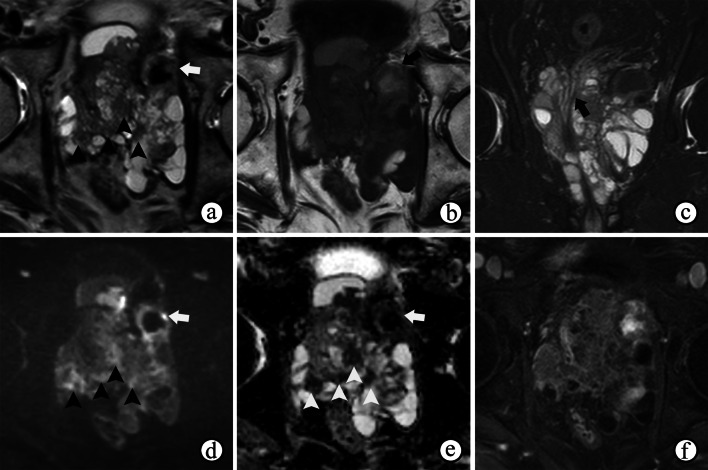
Mixed ductal and acinar adenocarcinoma in an 87-year-old man with dysuria for 12 months and a serum PSA level of 30.24 ng/mL. a–c Axial T2-, axial T1-, and coronal fat-suppressed T2-weighted imaging show a multilocular cyst mass occupying the prostate, periprostatic fat and bilateral seminal vesicles (black arrow on c), with heterogeneous signal intensity in cysts and multifocal solid areas (arrowheads on a), and a cyst with a thick wall and a mural nodule (arrows on a, b). d, e DWI at a b-value of 1000 s/mm^2^ and ADC maps show inhomogenously restricted diffusion of the solid areas (arrowheads) and the thick wall (arrows). f DCE imaging shows heterogeneous enhancement of the mass
